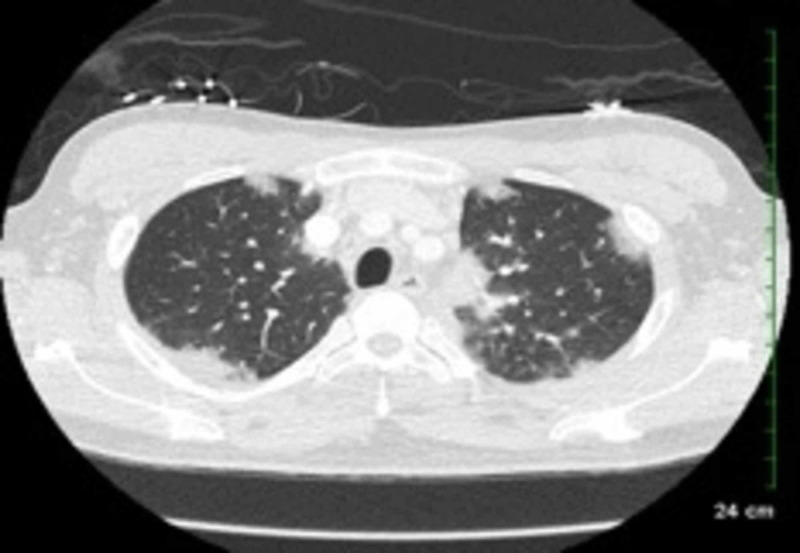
CT scan of the chest revealing bilateral lung infiltrates
